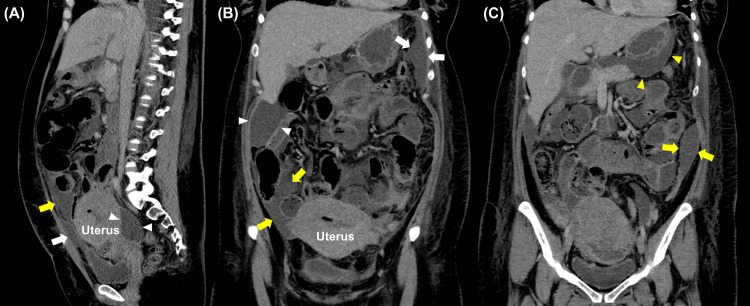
Contrast-enhanced abdominal CT image showing intra-abdominal abscesses extending into the peritoneal space The sagittal image (A) shows abscesses anterior to the uterus (white arrow), beneath the anterior abdominal wall (yellow arrow), and in the pouch of Douglas (white arrowheads). Coronal images (B, C) show abscesses in the left subphrenic space (white arrows), paracolic gutters (yellow arrows), hepatic flexure (white arrowheads), and omental bursa (yellow arrowheads).
